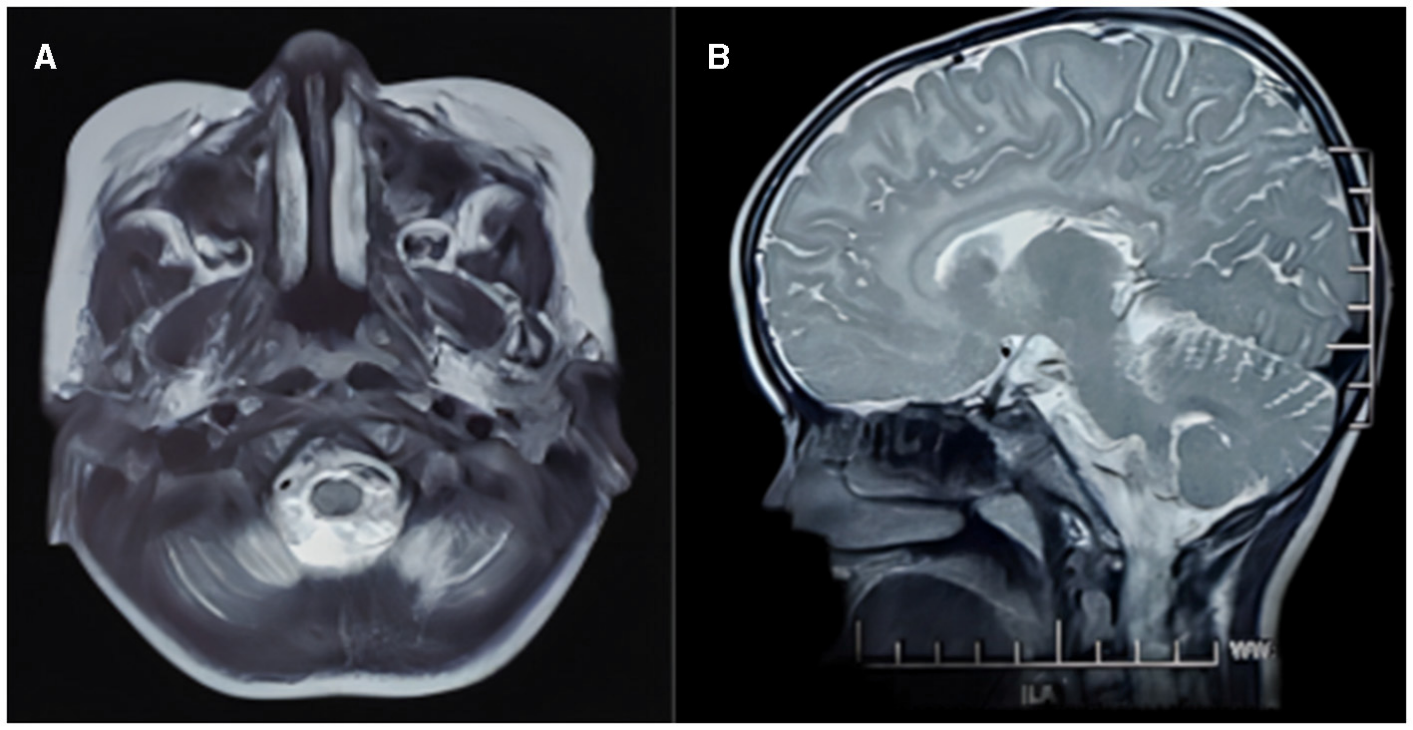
MRI images of a girl with POLR3-HLD (Patient 14), (A, B) at 5 years of age. The MRI image shows patchy T2WI hypersignal in the dentate nucleus of the cerebellum and thin corpus callosum with T2WI hypersignal.
